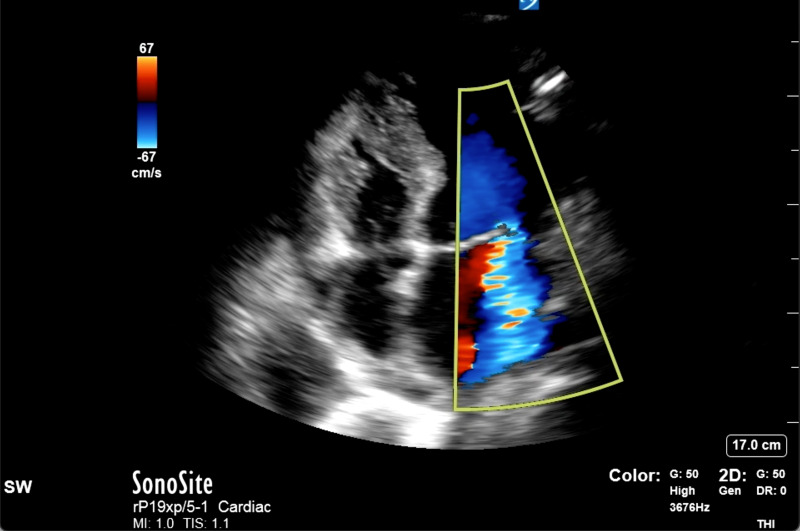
Cardiac point of care ultrasound (POCUS), apical four chamber view, demonstrating thin mitral valve leaflets and a large mitral regurgitant jet occupying half of the left atrium and extending to the posterior wall of the left atrium on color Doppler imaging.
